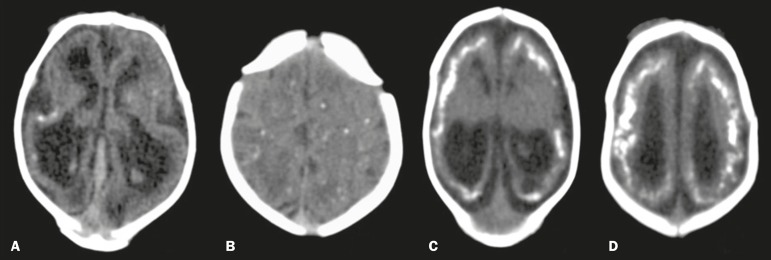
Non-contrast-enhanced CT scan showing cerebellar hypoplasia.
